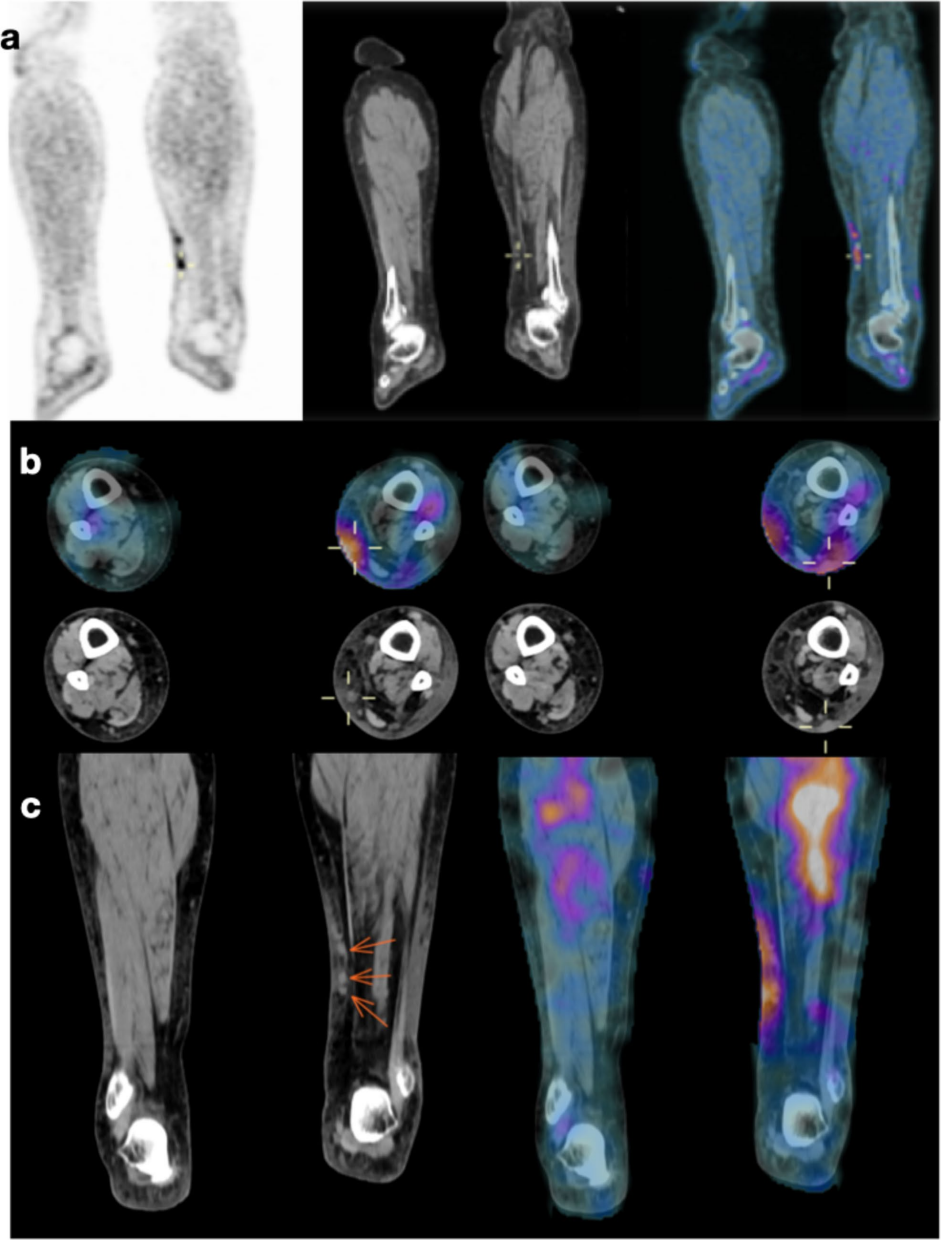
73-year woman with stage IIIC melanoma of the left calf melanoma and subcutaneous lesions: a) coronal views of PET image, CT image and fused [^18^F]FDG PET/CT image b) axial slices showing on top row fused [^99m^Tc]Tc-Tilmanocept SPECT/CT images at 1-h and CT images on bottom row c) coronal slices showing CT images with arrows pointing to subcutaneous lesions and fused [^99m^Tc]Tc-Tilmanocept SPECT/CT images at 1-h
